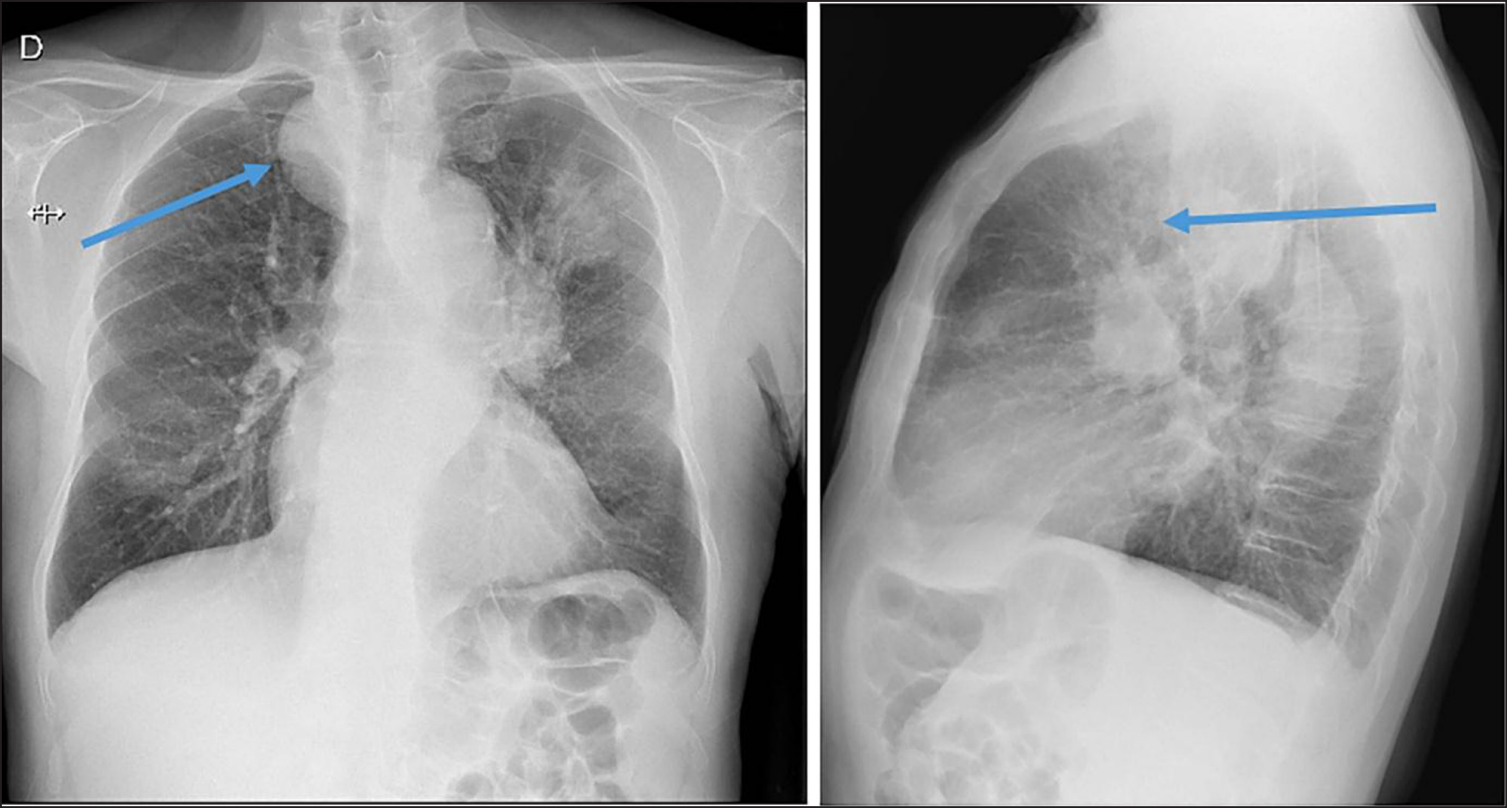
A chest computed tomography (CT) was performed the same day, confirming a pulmonary mass in the left upper lobe with lymphadenopathy in the aortopulmonary window. The right paratracheal lesion, however, proved to be an unsuspected aneurysm developed on an arteria lusoria (anatomical variant of right subclavian artery) named “Kommerell aneurysm.”
